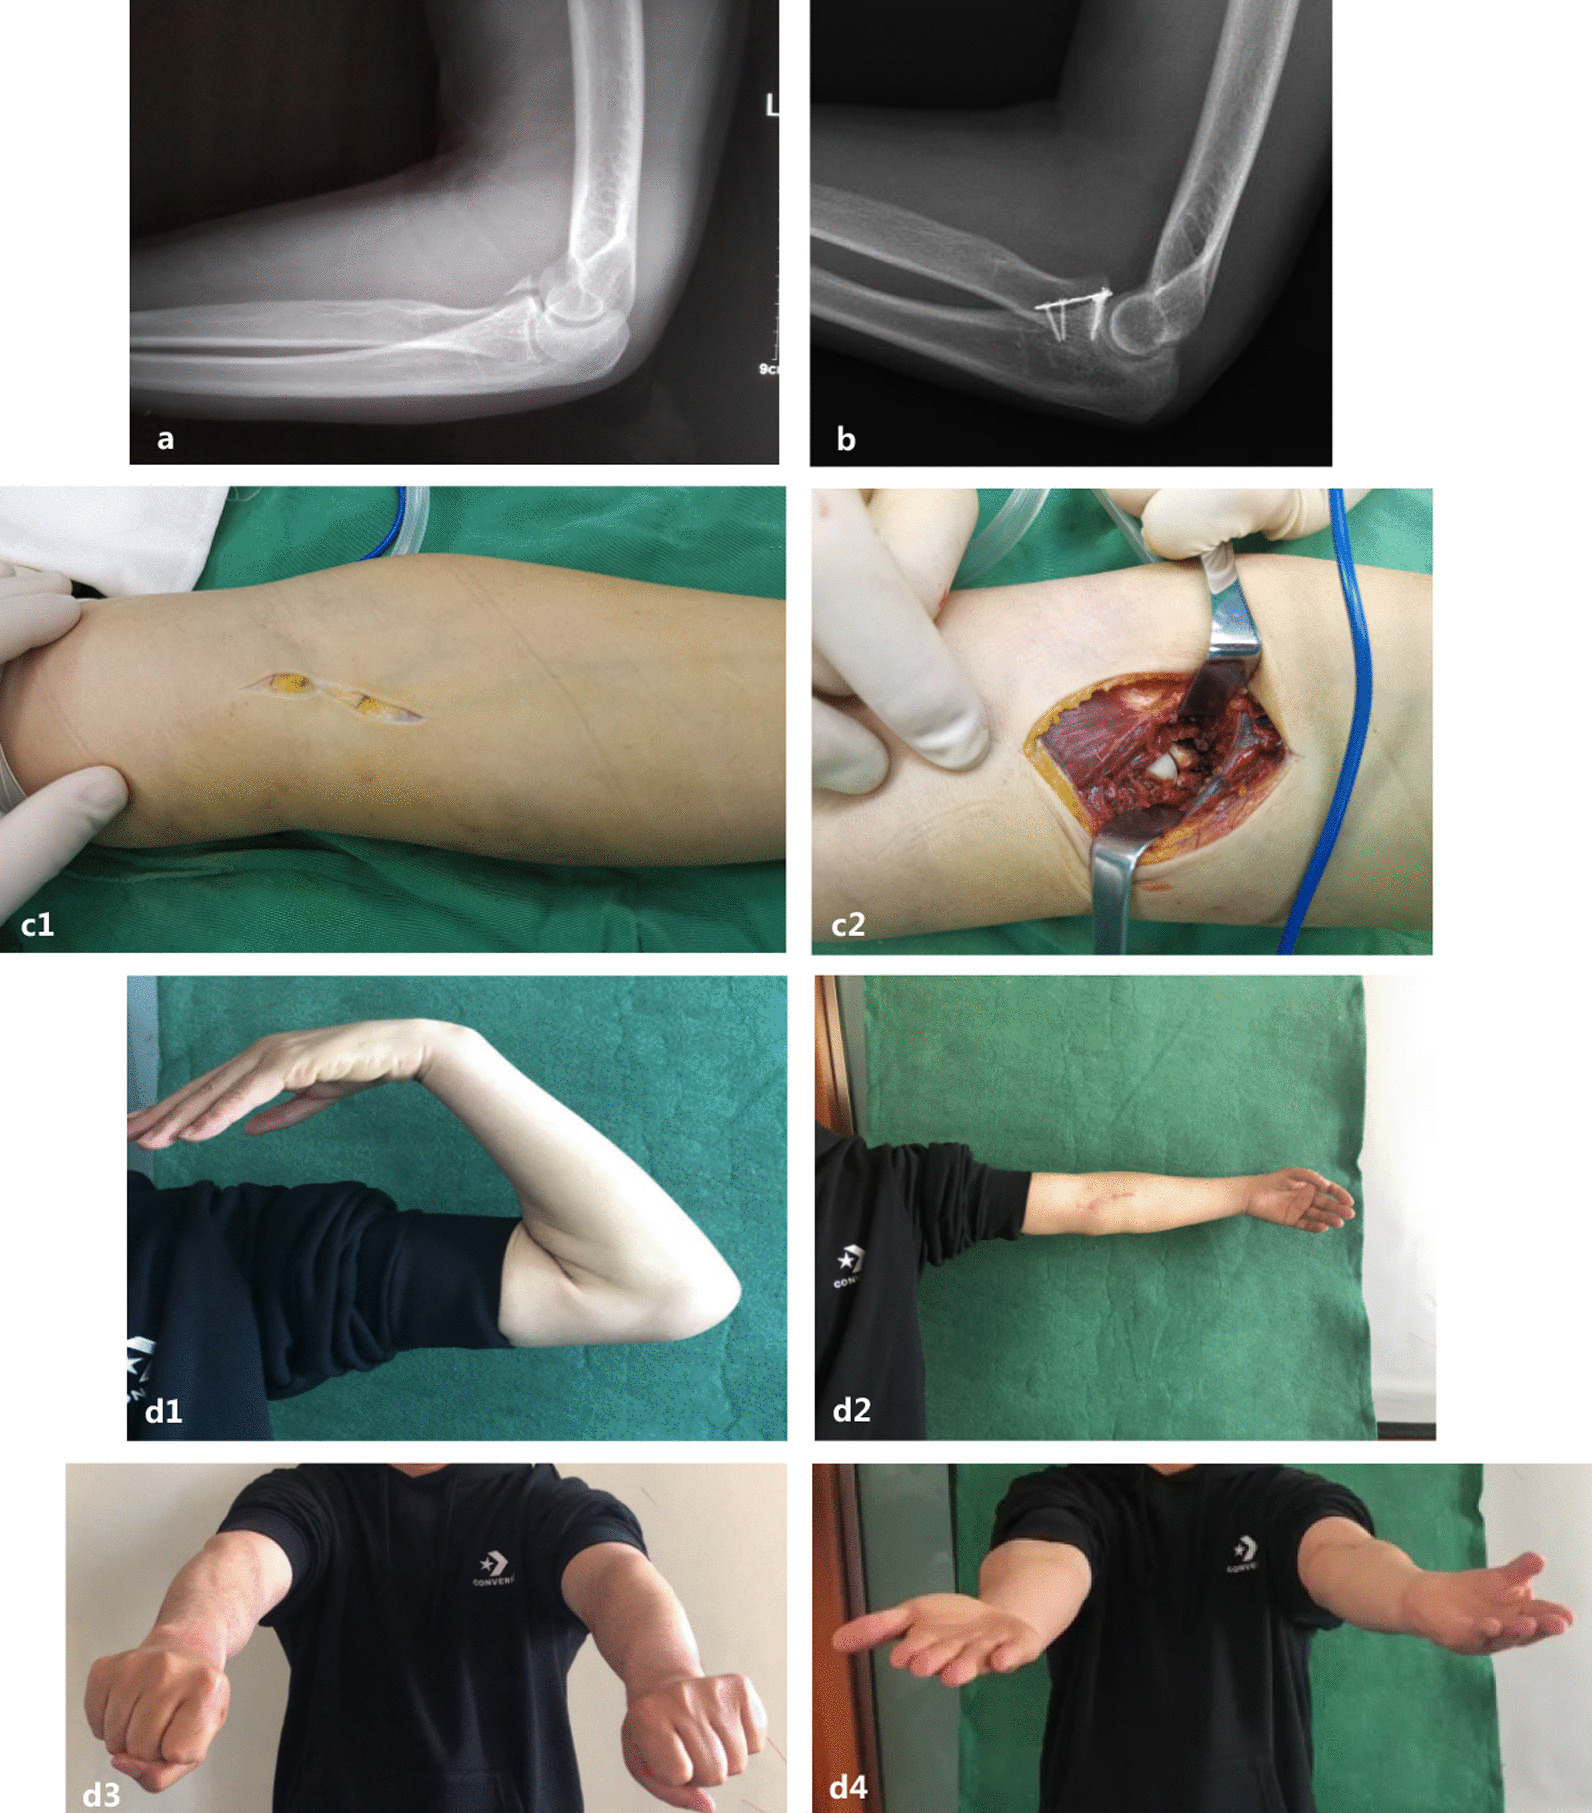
A 40-year-old man presented with a Regan and Morrey type II coronoid process fracture. Preoperative radiographs (a). Intraoperative exposure of the coronoid process through the anterior elbow approach (c). Solid union and good outcomes were achieved at the 8-month follow-up (b, d)
